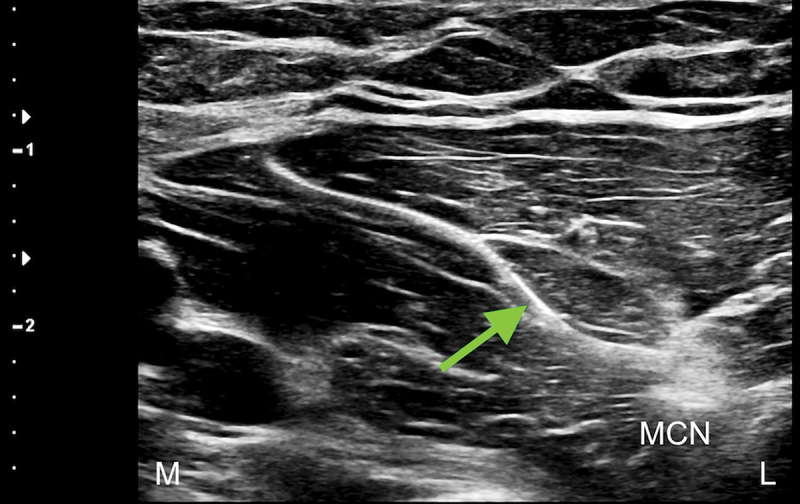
Ultrasound-guided medial-to-lateral in-plane percutaneous deployment of the peripheral nerve stimulator electrode, with visualization of the electrode tip just medial and superficial to the musculocutaneous nerve. Note: BBM = biceps brachii muscle; L = lateral; M = medial. Green arrow shows peripheral nerve stimulator electrode.
